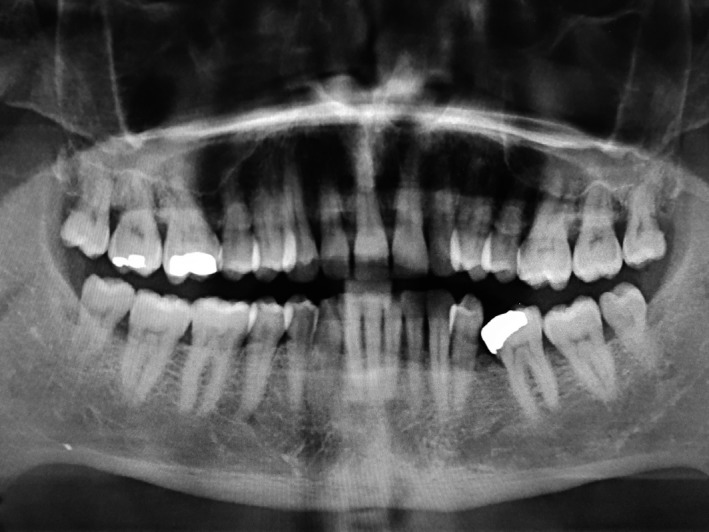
Panoramic radiograph showing multiple well‐defined radiopacities with a round/ovoid appearance all over the upper jaw
